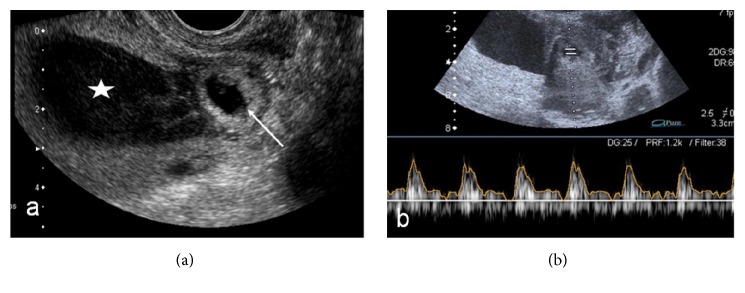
(a) A TVUS examination showing the gestational sac (arrow) near isthmus, close to previous cesarean section scar, and hemorrhagic fluid in endometrial cavity (star). (b) Spectral Doppler US showing the fetal pole and fetal heart rate in gestational sac.
